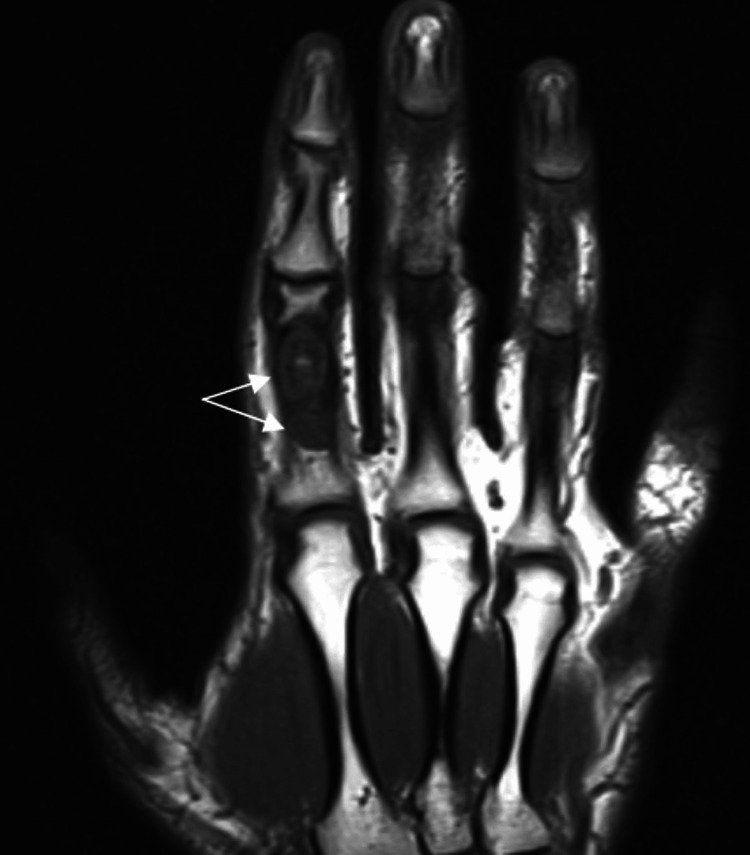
Preoperative MRI (T1 coronal) indicating double intraosseous lesions in the proximal phalanx of the index finger (white arrows).
